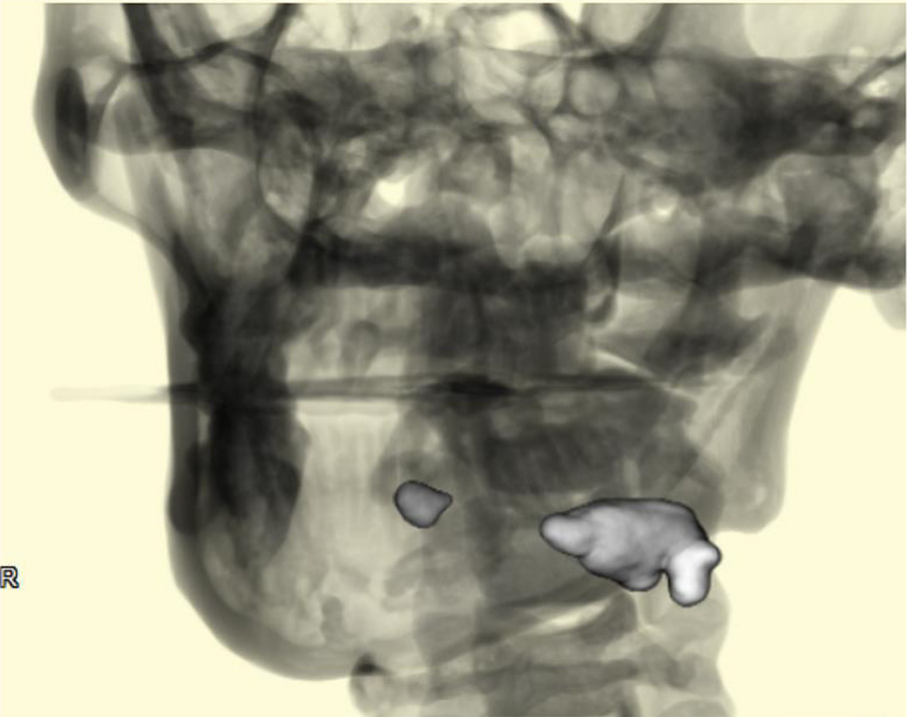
Three‐dimensional cone beam‐computed tomography reconstruction of the left submandibular parenchymal stones
